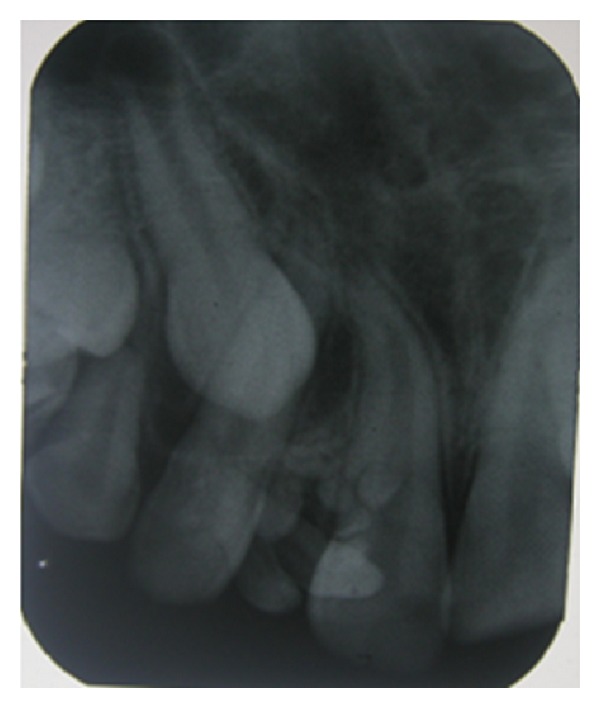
IOPAR showing radiographic appearance of odontoma.
